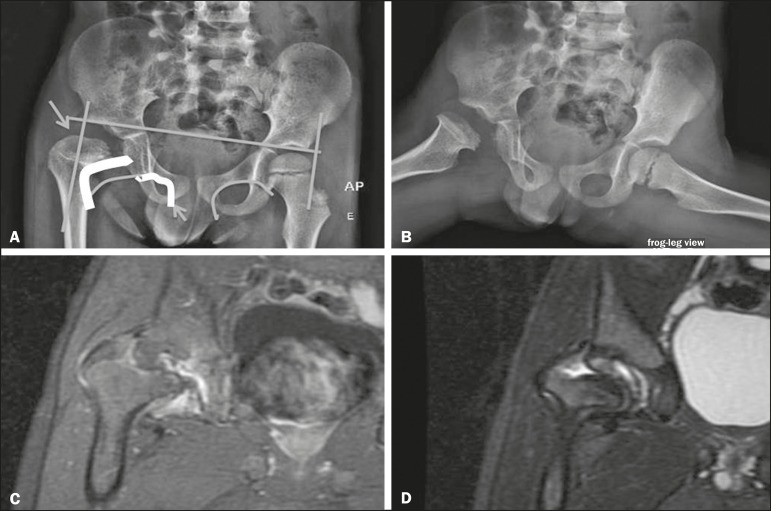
Dysplasia of the right hip in a 4 year-old male. Anteroposterior and frog-leg view X-rays (A and B, respectively), showing asymmetry of the hip joints, with right hip dysplasia. MRI of the right hip. Gadolinium-enhanced coronal, fat-suppressed T1-weighted sequence (C) and STIR sequence (D) showing a loss of sphericity, flattening, and superolateral subluxation of the right femoral head, with enlargement of the femoral neck and acetabular cavity, as well as joint effusion with signs of reactive synovitis.
